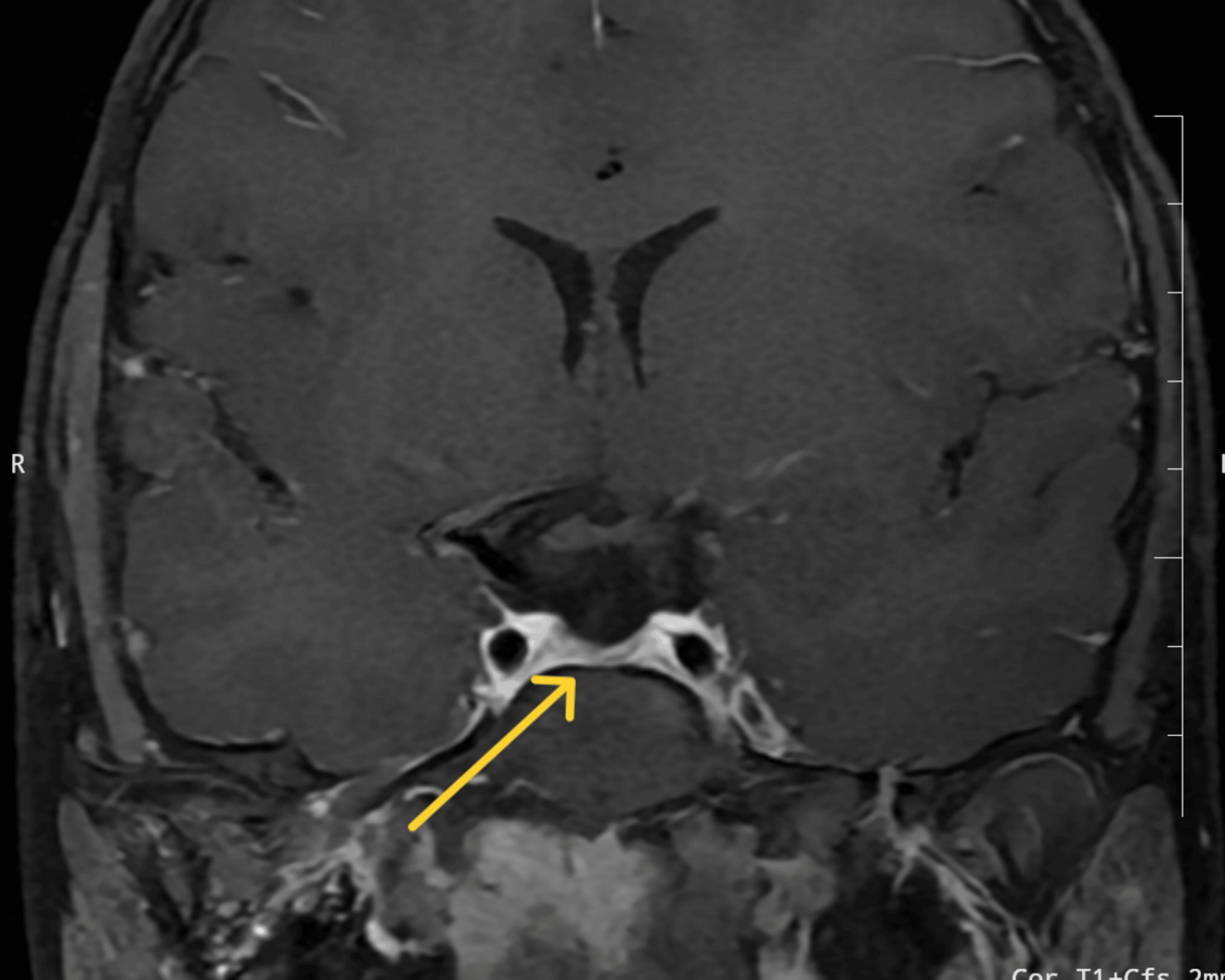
Contrast-enhanced magnetic resonance imaging of the sella turcica of Case 2 Contrast-enhanced magnetic resonance imaging of the sella turcica showing an ectopic posterior pituitary with a hypoplastic anterior pituitary (yellow arrow) measuring 1.9 mm in height, 4.6 mm in the anteroposterior dimension, and 6.8 mm in coronal diameter, and absent pituitary stalk.
